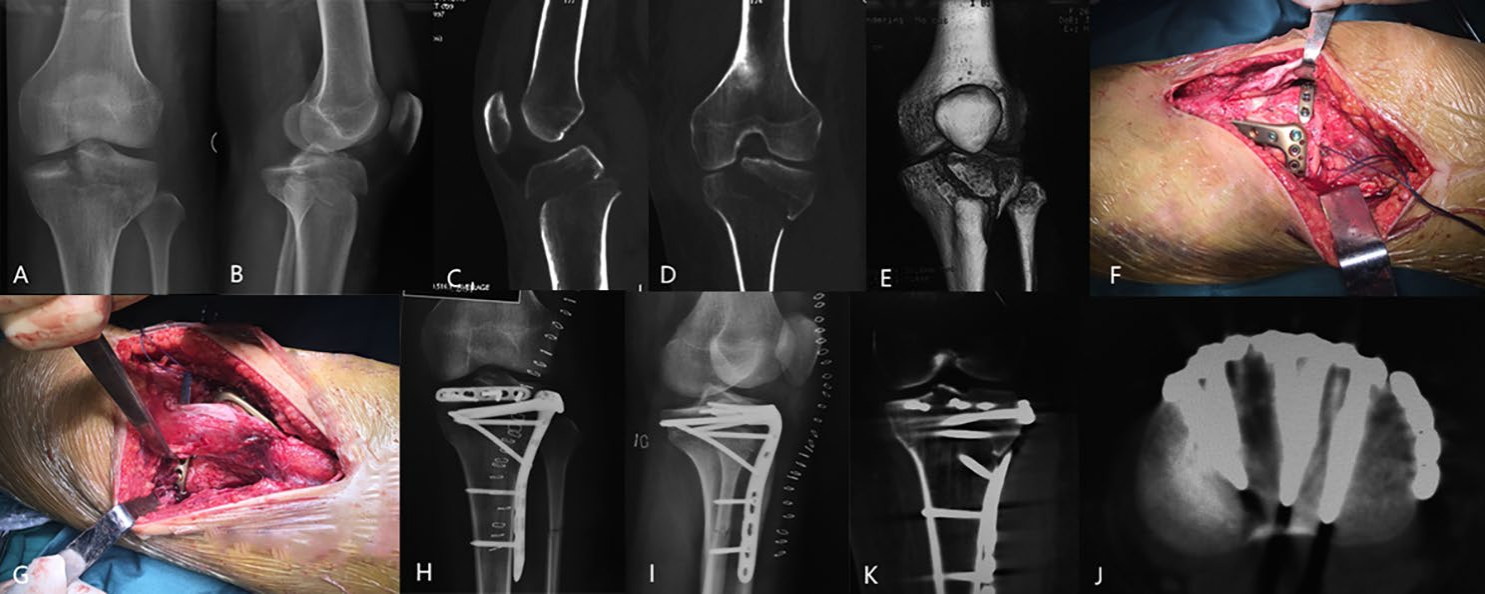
A 23-year-old female patient presented with lateral HTPF (A-E). Subsequently, open reduction and fixation were carried out using a combination of the rim plate and anterolateral locking plate (F-I). The screws of the rim plate were strategically placed by lifting both sides of the patella (F, G). Postoperative CT scans demonstrated the successful provision of sufficient support to the anterior plateau (J, K)
